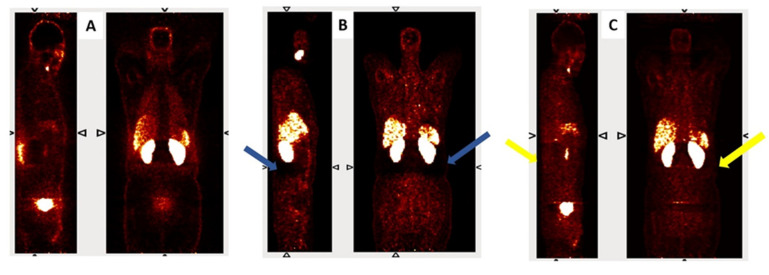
Representative coronal and sagittal views of (A) PET non-AC, (B) PET CT-AC, and (C) PET DL-AC-L1. Blue arrows indicate halo artifacts in the CT-AC image, while yellow arrows show partial correction in the DL-AC-L1 image.
